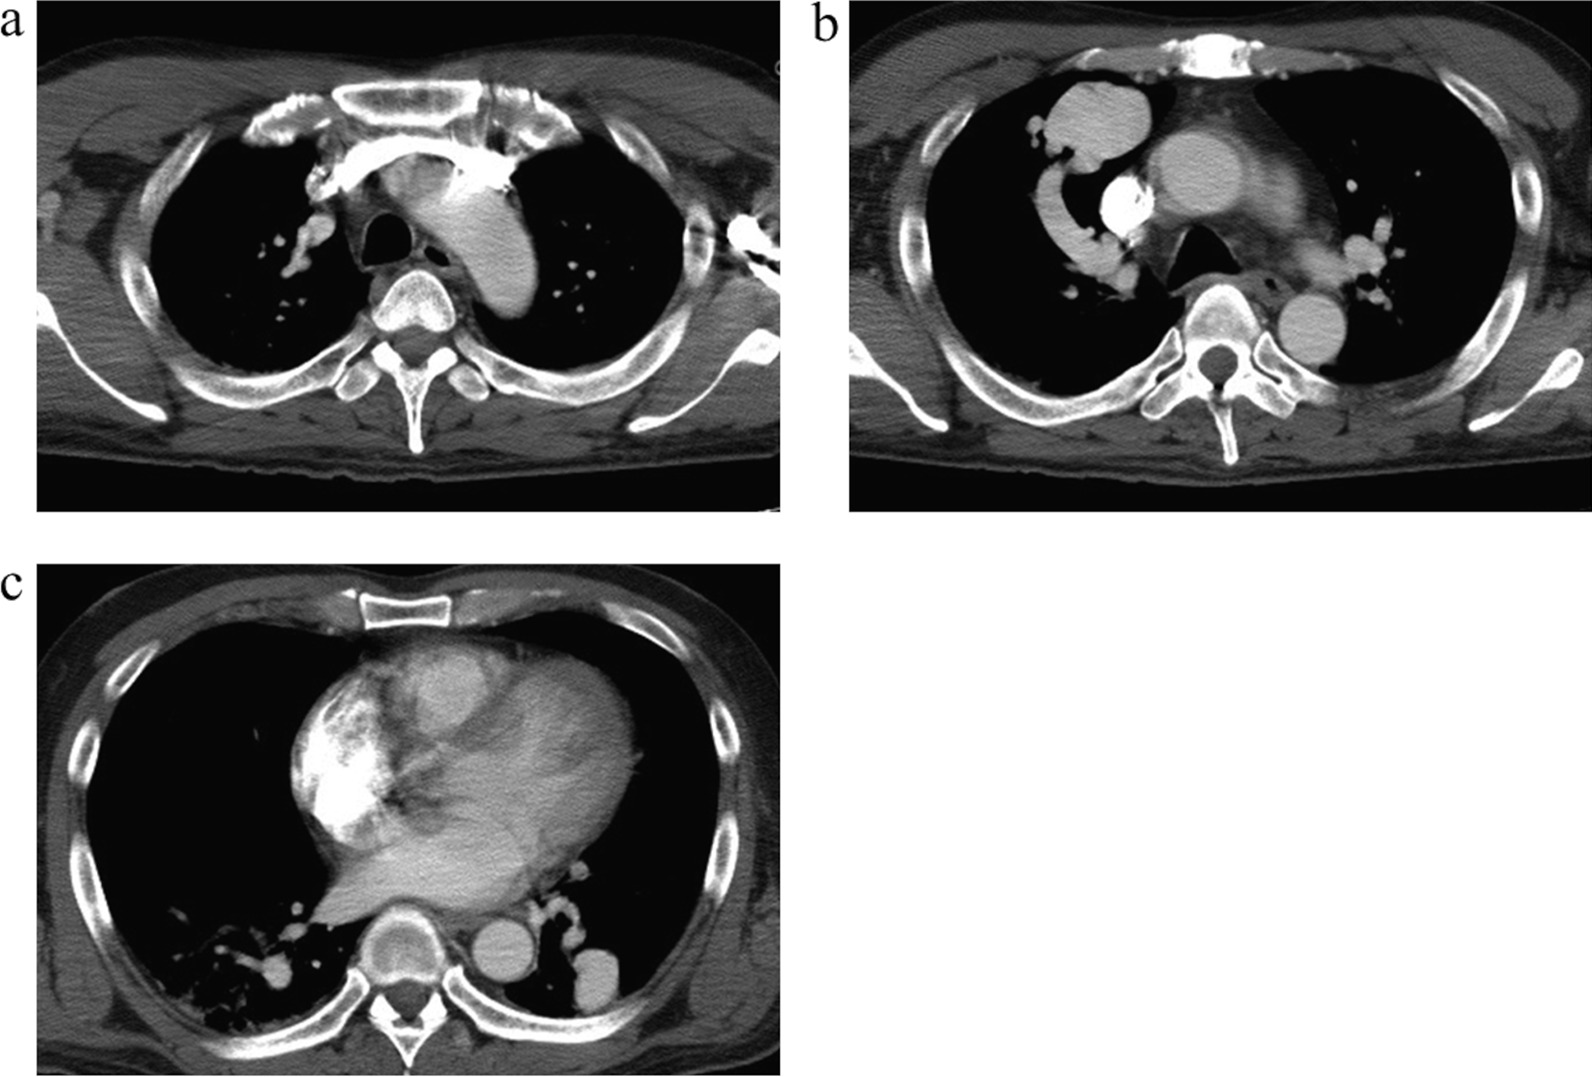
Enhanced chest computed tomography showed nodular lesions in right S1 (a), S3 (b) and left S10 (c)
